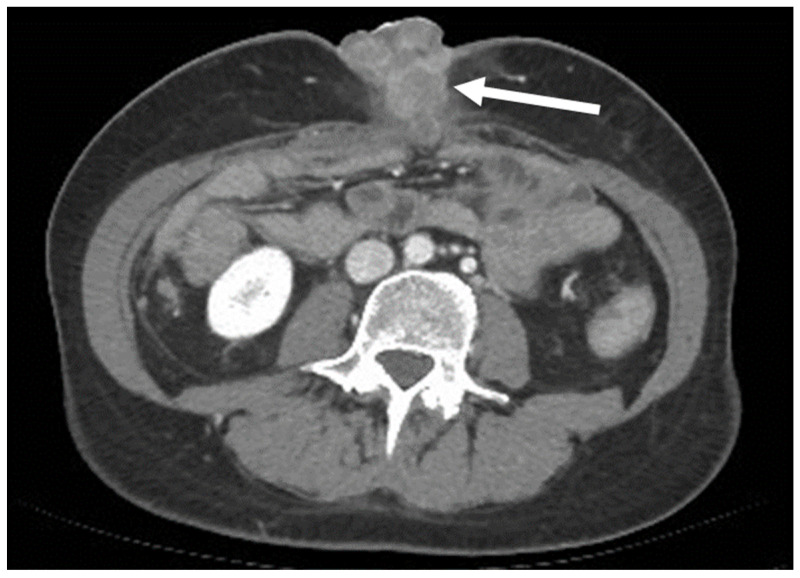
A 50-year-old woman with an umbilical mass. Axial, portal venous phase CT scan shows a collection of masses with heterogeneous, peripheral enhancement metastases along the navel (arrow). It was then demonstrated that the primary site of the tumor was an undifferentiated pancreatic carcinoma.
